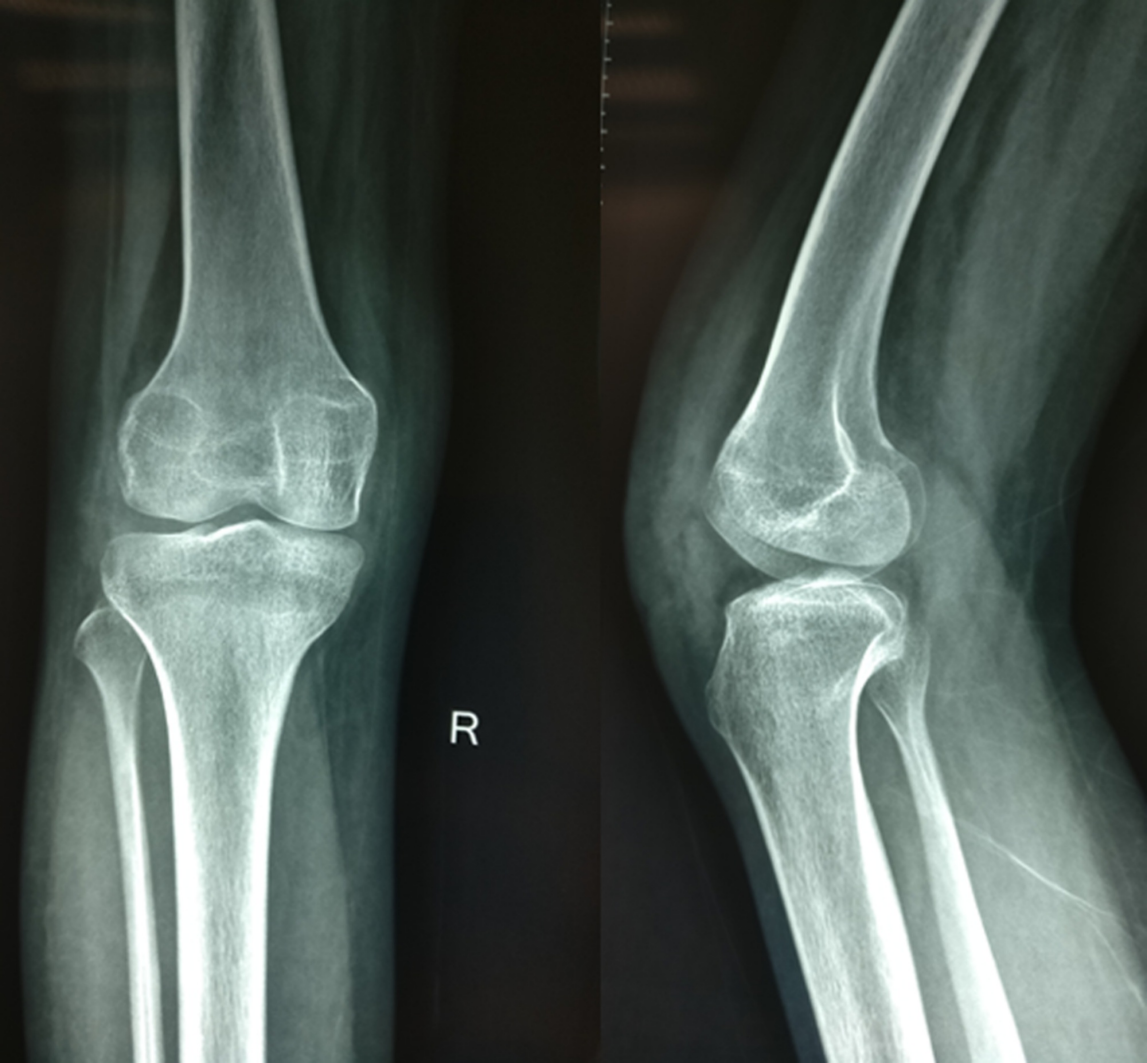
Follow up radiograph at 2-years shows absence of patella with no signs of recurrence
